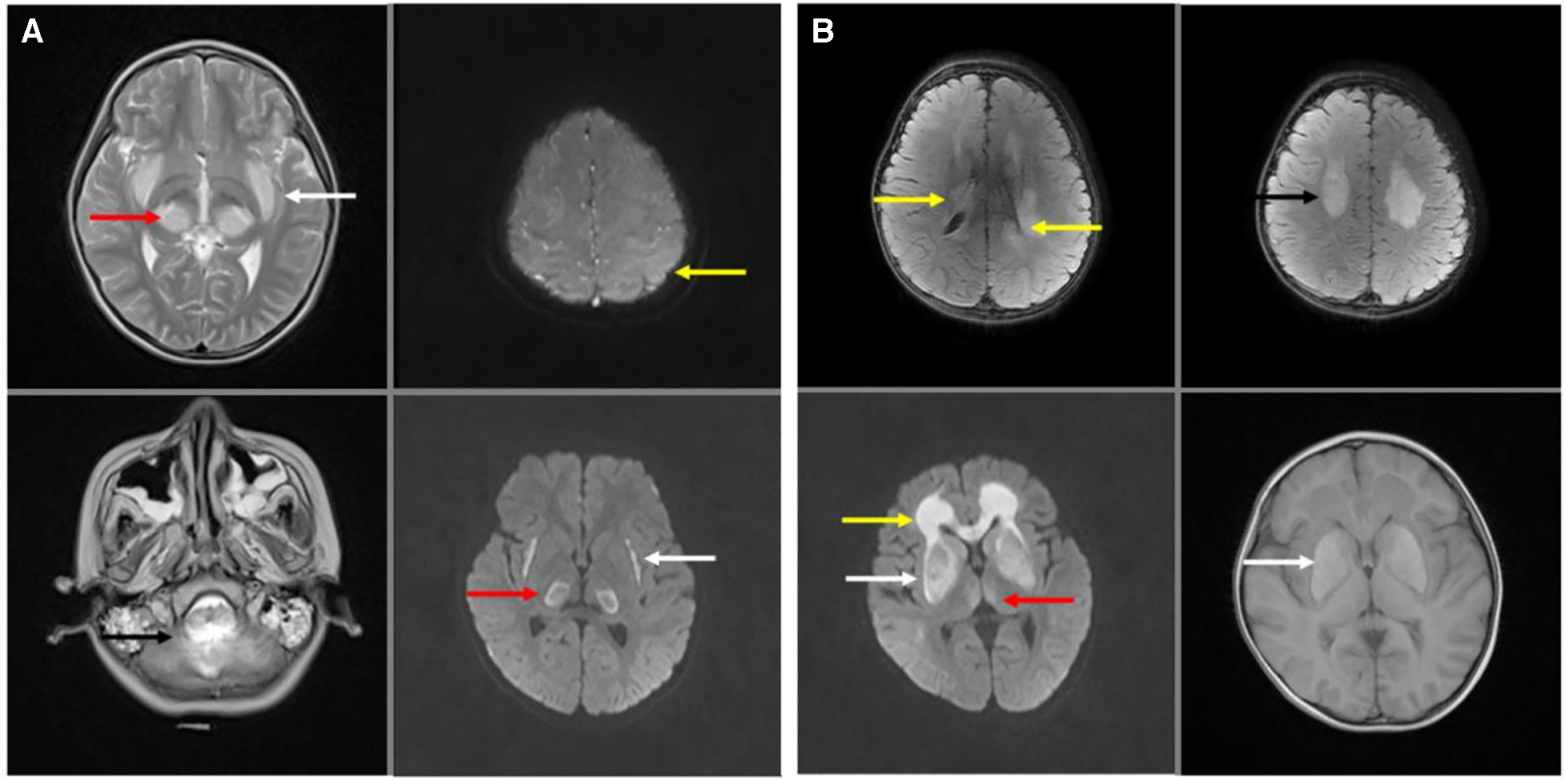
Brain nuclear magnetic resonance (MRI) of acute necrotizing encephalopathy. (A) Bilateral thalamic symmetrical damage in T2- weighted and T2-weighted flair (red arrow). Brain stem and cerebellum injured in T2- weighted (black arrow). The cerebral cortex was extensively oedematous with significant damage to the left parietal cortex (yellow arrow) in T2-weighted flair. External and internal capsule injury in T2-weighted flair (white arrow). (B) Bilateral thalamic symmetrical damage in T2-weighted flair (red arrow). Periventricular white matter damage in T2-weighted flair (yellow arrow). Centrum semiovale damaged in T2-weighted flair (black arrow). Basal ganglia damaged in T2-weighted flair and T1-weighted (white arrow).
